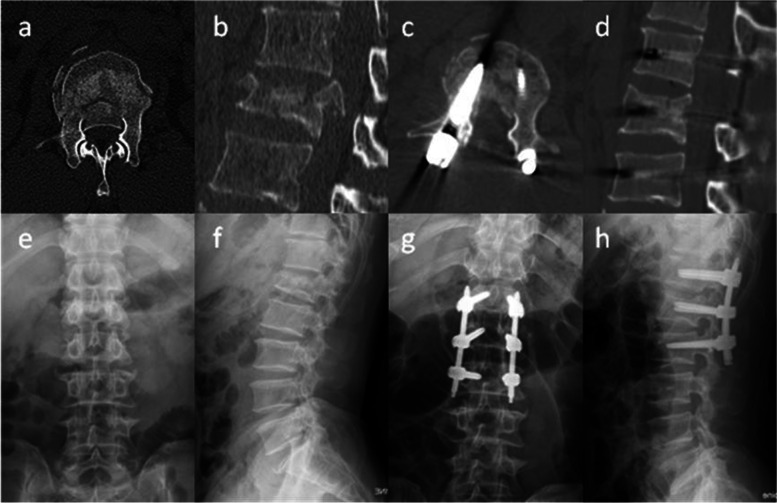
a–b Severe spinal canal encroachment as demonstrated by preoperative axial CT scan and sagittal reconstruction imaging. c–d Satisfactory improvement of spinal canal encroachment as demonstrated by postoperative axial CT scans and sagittal reconstruction imaging. e–f Lumbar AP and lateral X-ray preoperatively. (g–h) Lumbar AP and lateral X-ray postoperatively
